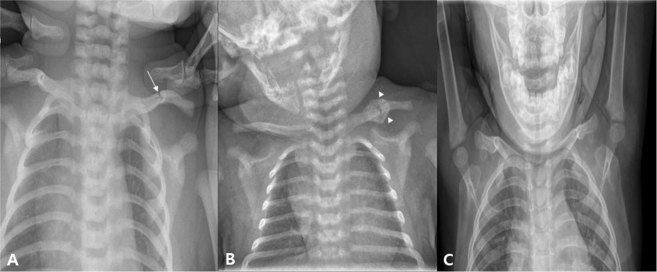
An infant who visited our hospital for left torticollis. In ultrasound examination, there was muscular fibromatosis in right SCM. (A) At 4 days after birth, left clavicular fracture was seen (arrow). (B) Follow-up plain radiograph at 21 days after birth. A callus formation was seen around fracture site of left clavicle (arrowhead). For clavicular fracture, only a conservative treatment was performed except stretching exercise and home education for right CMT. (C) Follow-up plain radiograph at 22 month after birth. Callus formation was disappeared, and it is difficult to find the difference between left and right clavicle. CMT; congenital muscular torticollis, SCM; sternocleidomastoid.
